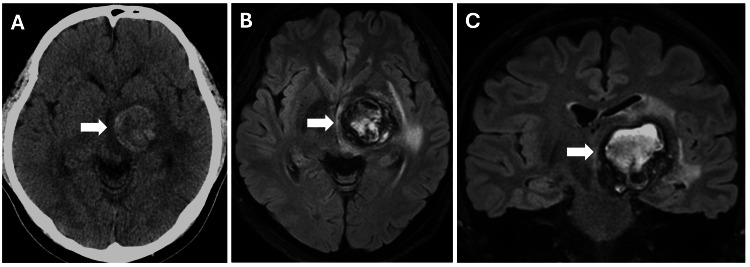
Initial imaging studies (Case 1) Computed tomography on admission demonstrating a left thalamic hemorrhage with a maximum diameter of 3 cm (A, arrow). Fluid-attenuated inversion recovery magnetic resonance imaging showing a reticulated pattern of mixed hyperintensity, hypointensity, and mild edematous changes around the hemorrhage (B and C, arrows).
